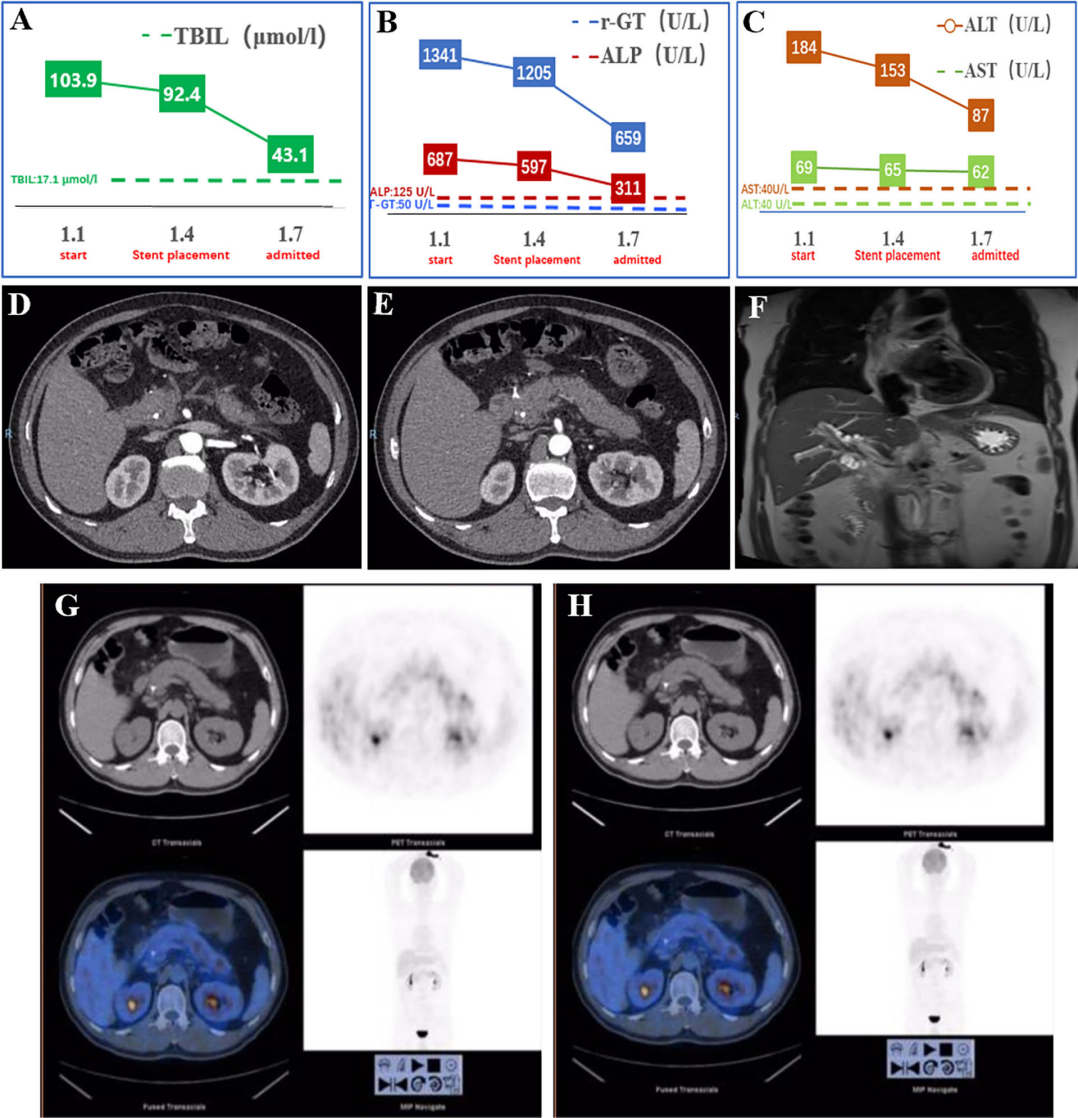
Examination results before and on admission. (A–C) The TBIL, γ-GT, ALP, AST, and ALT changing curve of the patient. The measuring frequency was every 3 days in the local hospital. (D, E) The abdominal and contrast-enhanced CT scan showed a hyperdense mass in the common bile duct (biliary stent). The pancreatic parenchyma is plump, and adipose tissue around the pancreas is cloudy, which suggested pancreatitis. (F) MRCP showed stenosis of the common bile duct. (G, H) PET-CT did not show any signs of malignancy. TBIL, total bilirubin; γ-GT, γ-glutamyl transpeptidase; ALP, alkaline phosphatase; AST, aspartate aminotransferase; ALT, alanine aminotransferase.
